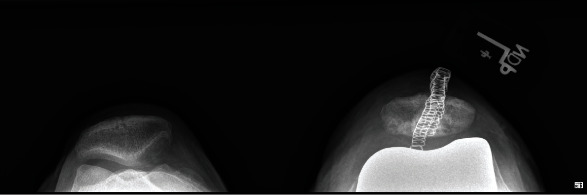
Postop merchant knee X-ray.
